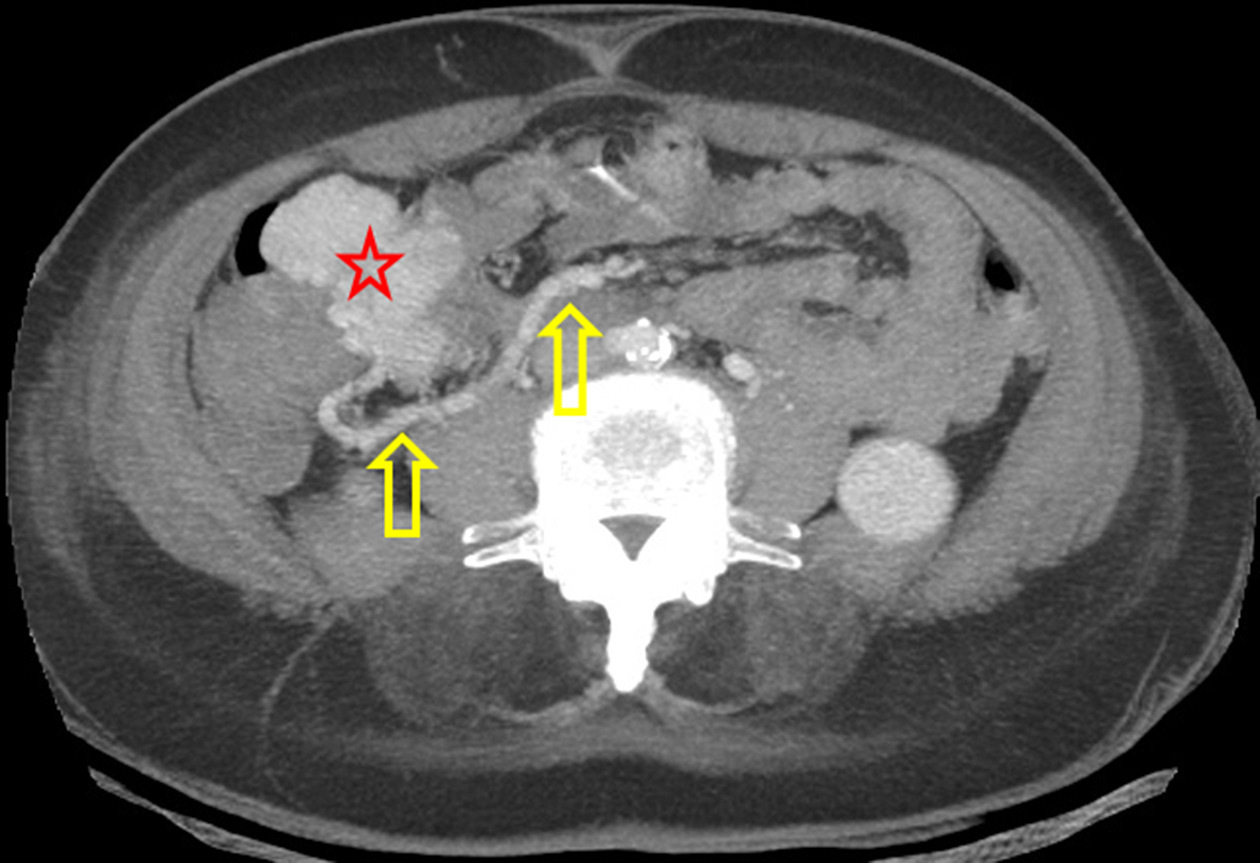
Maximum intensity projection of axial contrast-enhanced CT scan in arterial phase. Exophytic ileal mass (red star and drained by aberrant hypertrophic vascular structure originating from the portal vein (yellow arrows).
